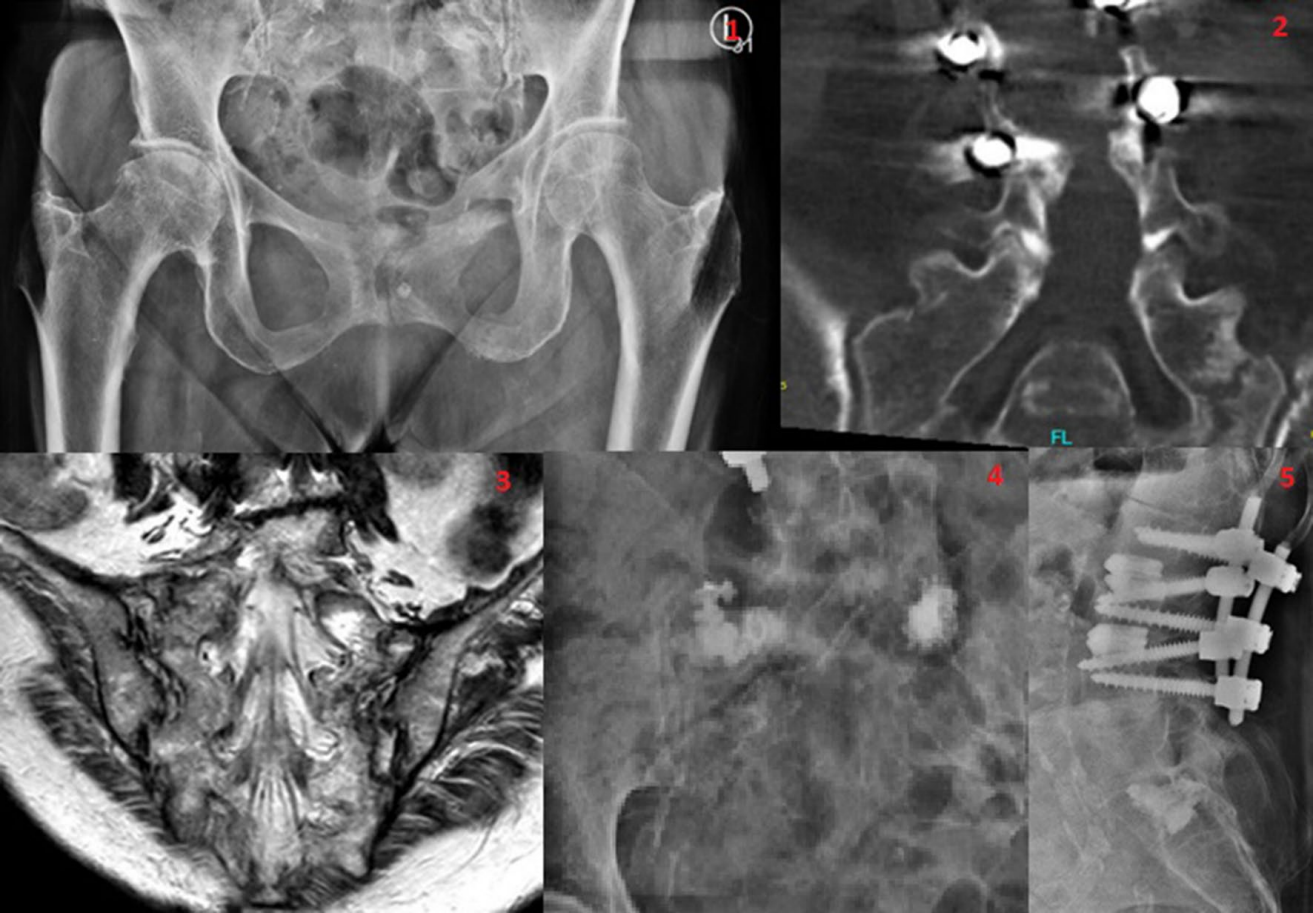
Imaginary findings of one of the patients with a bilateral sacral insufficiency fracture being the first an anterior–posterior plane of the X-ray preoperatively, the second a CT-scan in anterior–posterior plane preoperatively, the third an MRI in anterior–posterior plane preoperatively, the forth an anterior–posterior plane of the X-ray postoperatively and the fifth an lateral plane of the X-ray postoperatively.
